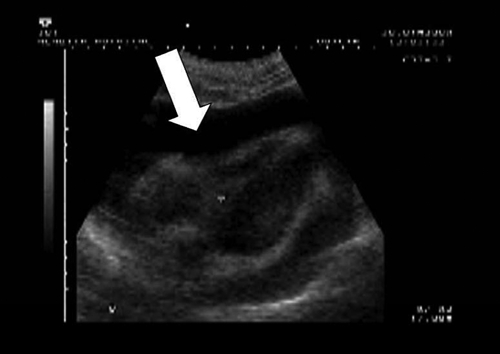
Ultrasound at presentation for the patient, showing large pericardial effusion and right ventricular collapse.
